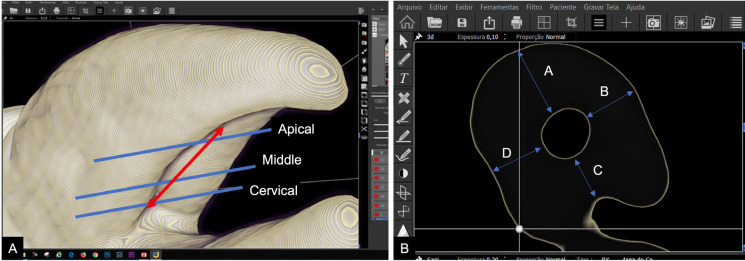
(A) 3D reconstructions showing the measurements sites: 1 mm coronal to the groove (cervical), at the groove’s deepest point(middle), and 2 mm apical to the groove (apical). (B) CBCT image demonstrating the dentin thickness measurement areas on the buccal root using the e-volDX software filter. The measured root walls are identified as follows: A- buccal, B - Mesial, C - Palatal and D - Distal.
